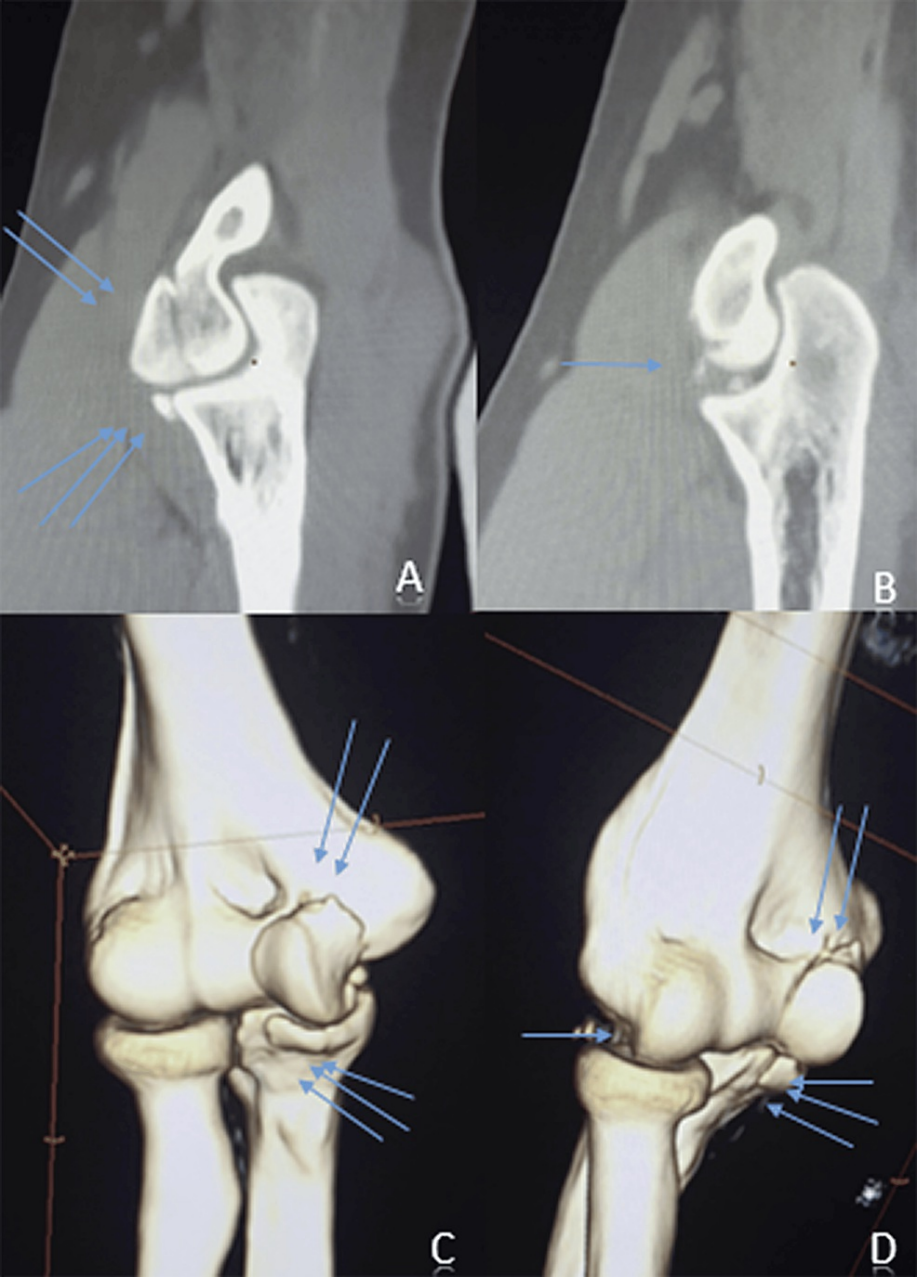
CT scans of the elbow. A and B (sagittal sections), C and D (3D reconstructions) showing intra-articular fragments (one blue arrow), the trochlear fracture (two blue arrows), and avulsion of the coronoid process (three blue arrows)
